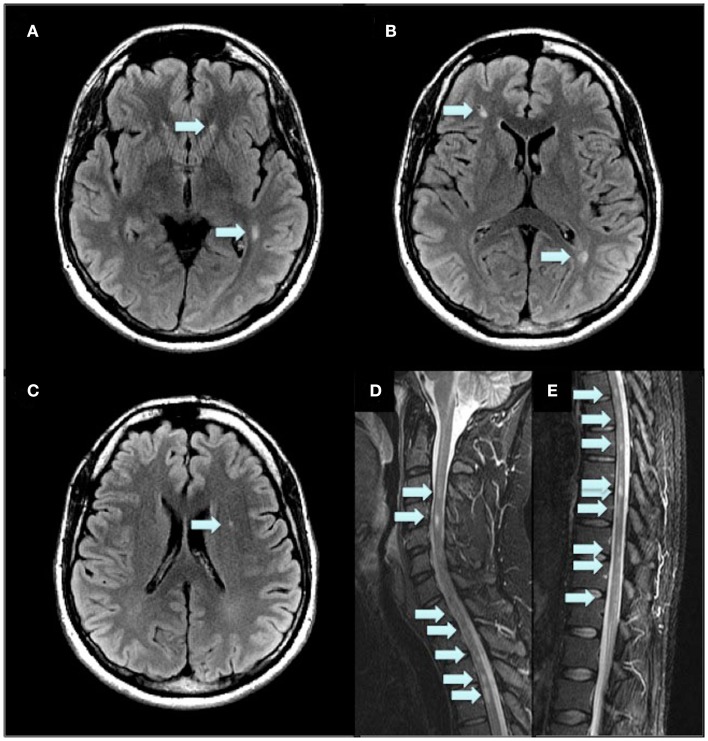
Initial MRI 2D Flair of the brain (A–C) and FSTIR of the spinal cord (D,E) at the time of MS diagnosis. Arrows indicate MS lesions.
